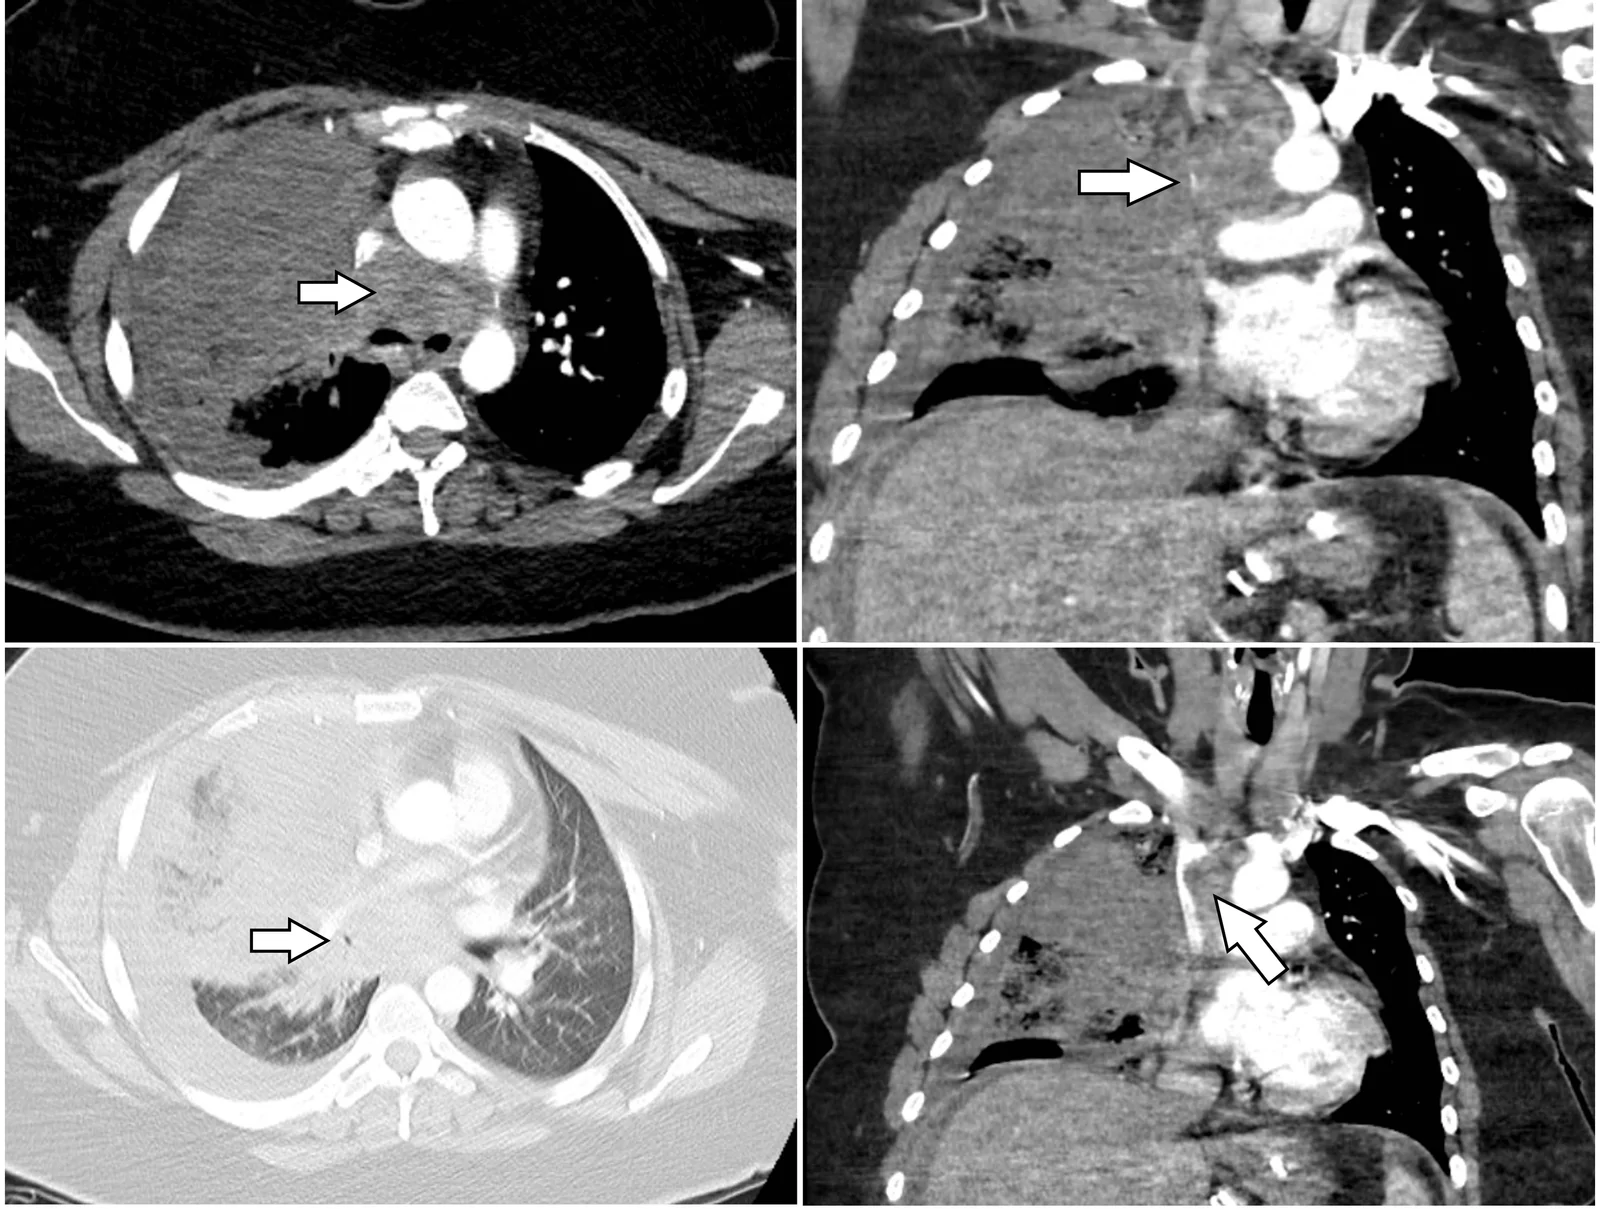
Contrast-enhanced computed tomography of the chest demonstrates a large right superior mediastinal mass. The top left (axial) and top right (coronal) images show the bulky mediastinal mass (white arrows) causing marked compression and near-complete effacement of the superior vena cava (SVC). The bottom left (axial) image demonstrates severe compression of the right mainstem bronchus (white arrow). The bottom right (coronal) image demonstrates near-complete SVC obstruction secondary to extrinsic compression by the mediastinal mass (white arrow).
